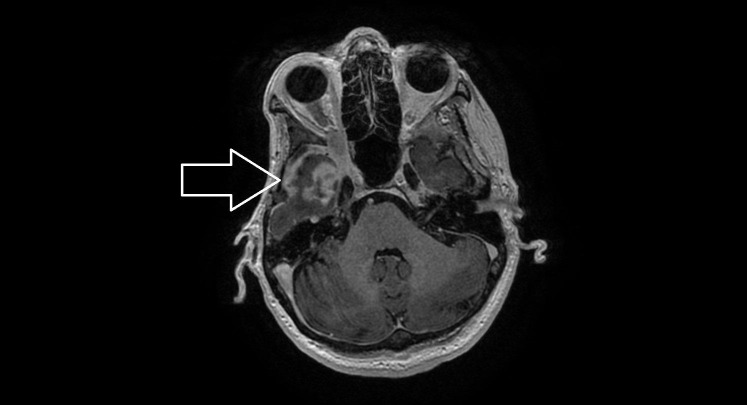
The magnetic resonance imaging of the orbital metastases causing compressive neuropathy.
